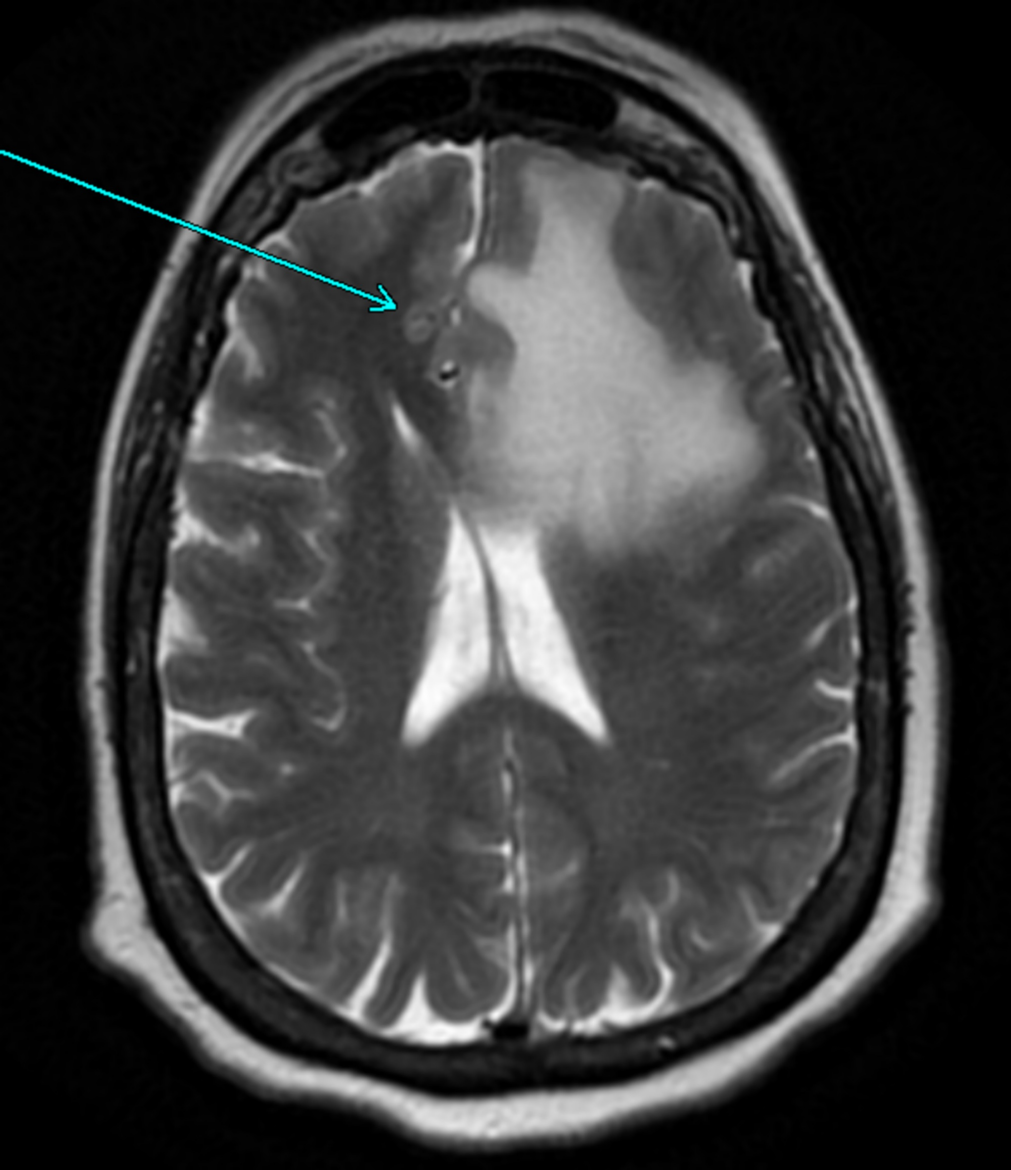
MRI brain with contrast axial view T2 sequence shows left frontal lobe growth with midline shift to right.
